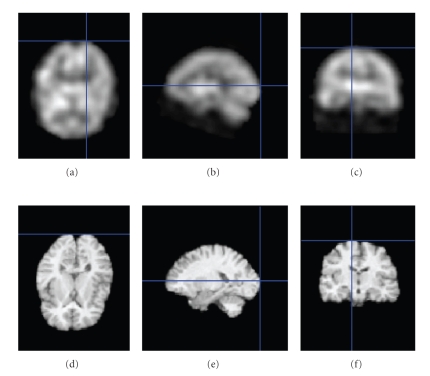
Examples of registration of the MR volume to the SPECT volume (using registration method described in Section 3.1). (a), (b), (c): Axial, sagittal, and coronal view of a SPECT volume. (d), (e), (f): Axial, sagittal, and coronal view of a registered MR volume.
